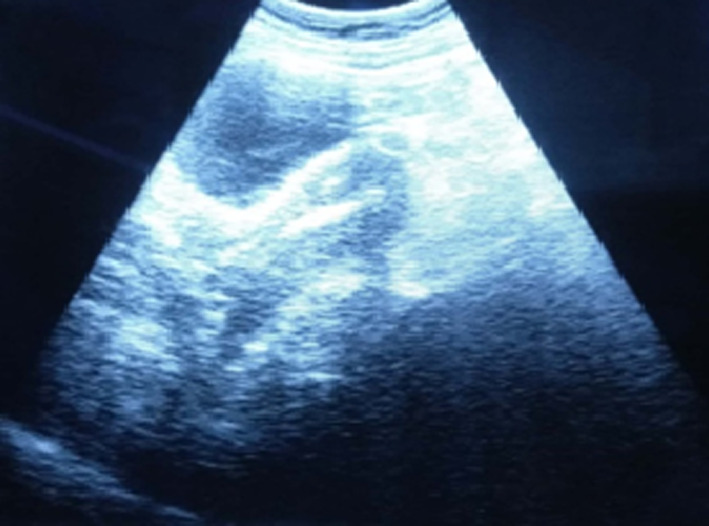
Ultrasonography image showing IUD in situ
